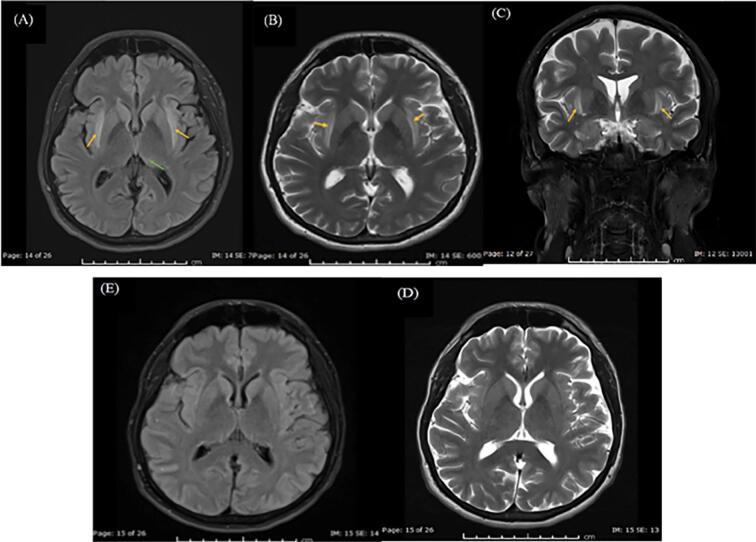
MRI head. Axial Fluid Attenuation Inversion Recovery (FLAIR), [B-C] Axial and Coronal T2-weighted images: Bilateral symmetric external and extreme capsules high T2-signal intensity with sparing of the intervening claustrum (orange arrows) bilaterally and subtle focus of high signal intensity left thalamus (Green arrow). 18 days follow up MRI [D] FLAIR images [E] T-2 weighted images: Significant regression with near total resolution of previously seen bilateral symmetric external and extreme capsules high T2-signal intensity as well as the subtle focus of high signal intensity at left thalamus.
